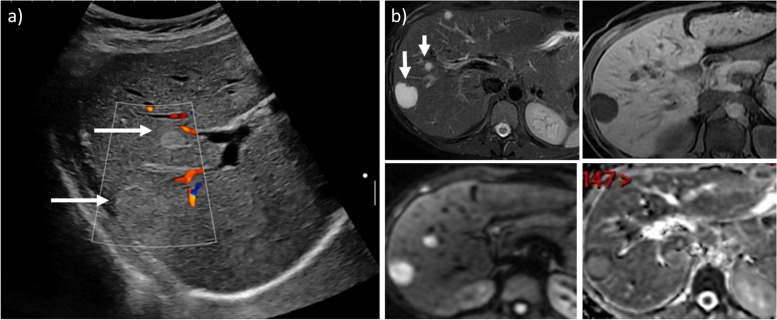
During a routine US (a), hyperechoic hepatic lesions (arrows) were seen in a 37-year-old pregnant woman. These lesions showed no vascularization on Doppler US nor stiffness, and the liver had no signs of chronic liver disease, so they were classified as hemangiomas. Months later, one lesion slightly grew, and an unenhanced MR was performed (b), showing high T2 signal with low T1 signal (upper row) and no diffusion restriction (lower row), thus confirming the diagnosis of hemangiomas
